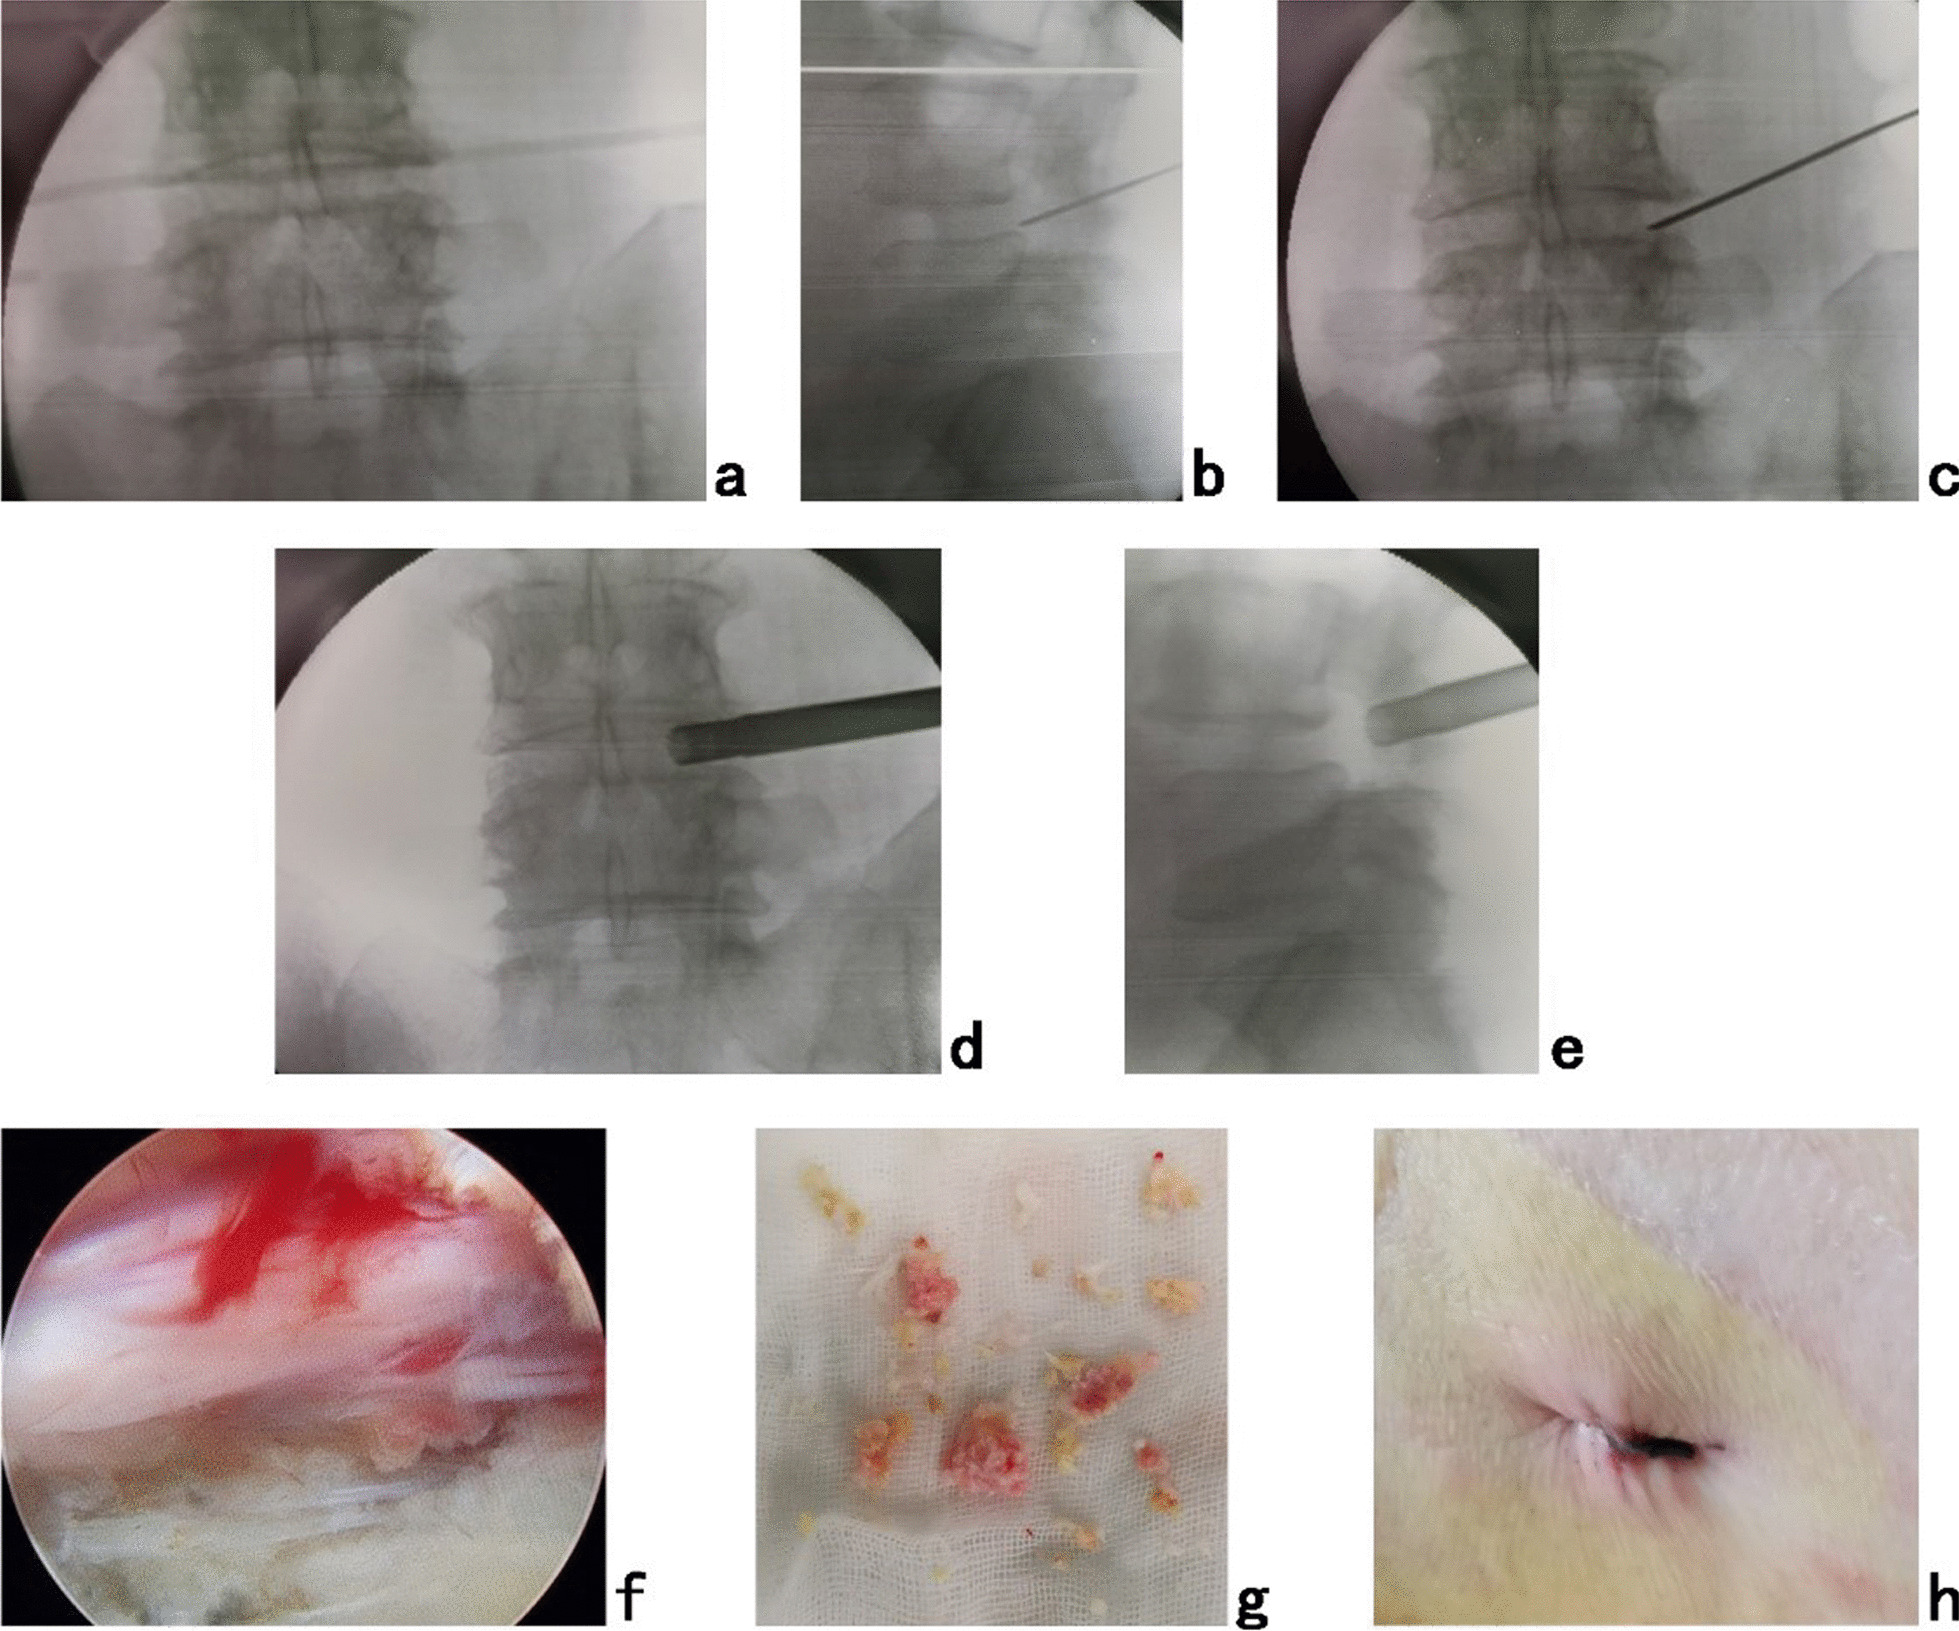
The patient had the pain of right lateral buttock, posterolateral thigh, lateral calf, and dorsal foot, which indicates L5 nerve root is involved, and the culprit segment was predicted as L4/5. PTES was performed under local anesthesia for L4/5. A transverse line bisecting L4/5 disc was drawn along the metal rod which was placed transversely across the center of the target disc on a posteroanterior C-arm view. After successful puncture, the C-arm view was taken to ensure that the needle reached the target. The tip of puncture needle was in the intracanal area close to the posterior wall of the disc on b lateral X-ray and near the lateral border of the pedicle on c posteroanterior X-ray. During press-down enlargement of foramen, when resistance disappeared, the tip of reamer should exceed the medial border of the pedicle on d posteroanterior C-arm view and reach close to the posterior wall of the target disc on e lateral C-arm view. Under f endoscopic view, the compressed nerve root was freed after g the hypertrophic ligmentum flavum and herniated disc were removed. h The stab incision of 8 mm was closed after confirming the efficacy
